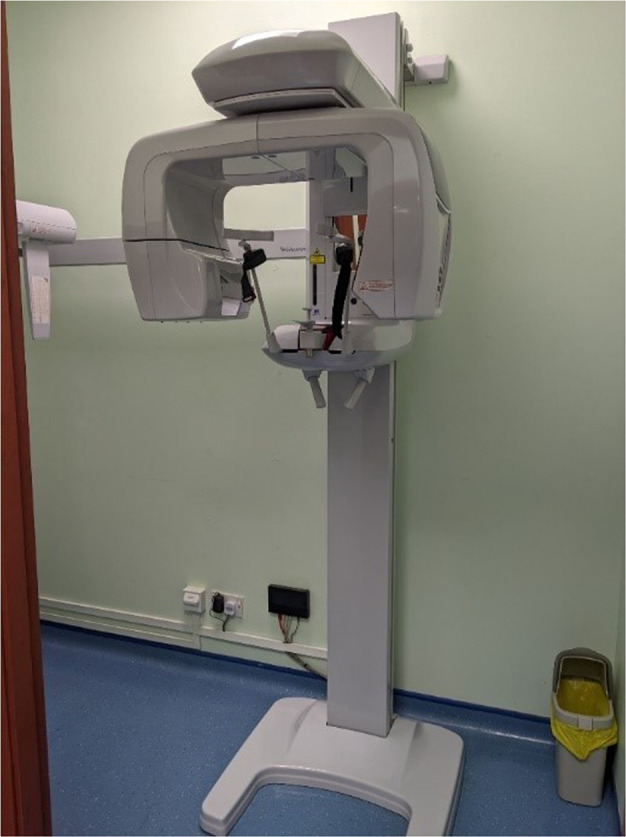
CBCT scanner. CBCT scanner used in the study which is located in the National Dental Centre, Brunei Darussalam.
